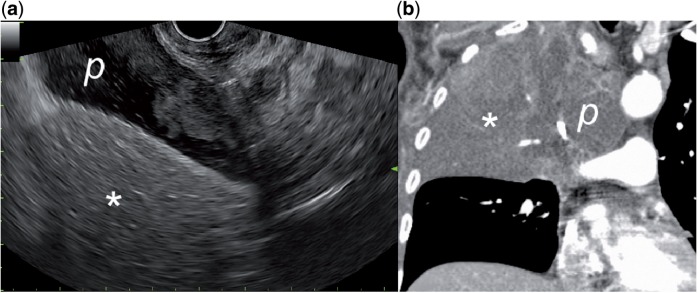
(a) Linear endoscopic ultrasound view of a large pleural effusion (p) surrounding an area with tissue-like pattern resembling that of liver (asterisk). The endoscope is positioned at the upper oesophagus and orientated towards the right lateral wall (clockwise direction). (b) Computer tomography image of the same anatomical region showing an atelectatic superior upper lobe surrounded by the pleural effusion.
